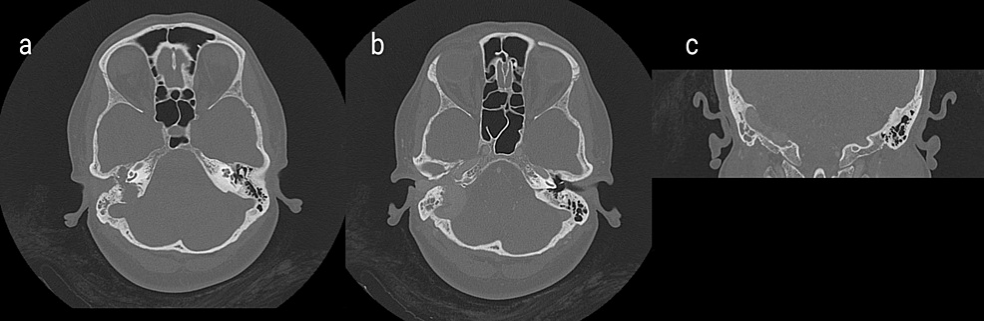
Contrast-enhanced computed tomography (CT) findings A heterogeneous soft tissue mass about 2.5x2x4 cm. (a, b) axial CT showing eroded right jugular foramen and expanding through the floor of the middle ear to the right external auditory meatus. In close proximity to the right sigmoid sinus and internal jugular vein, (c) coronal CT: intracranial extension of the lesion.
